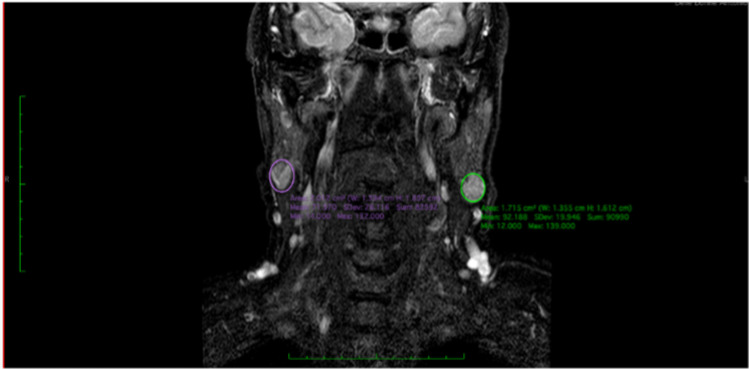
MR images of a synchronous contralateral WT in coronal projection
